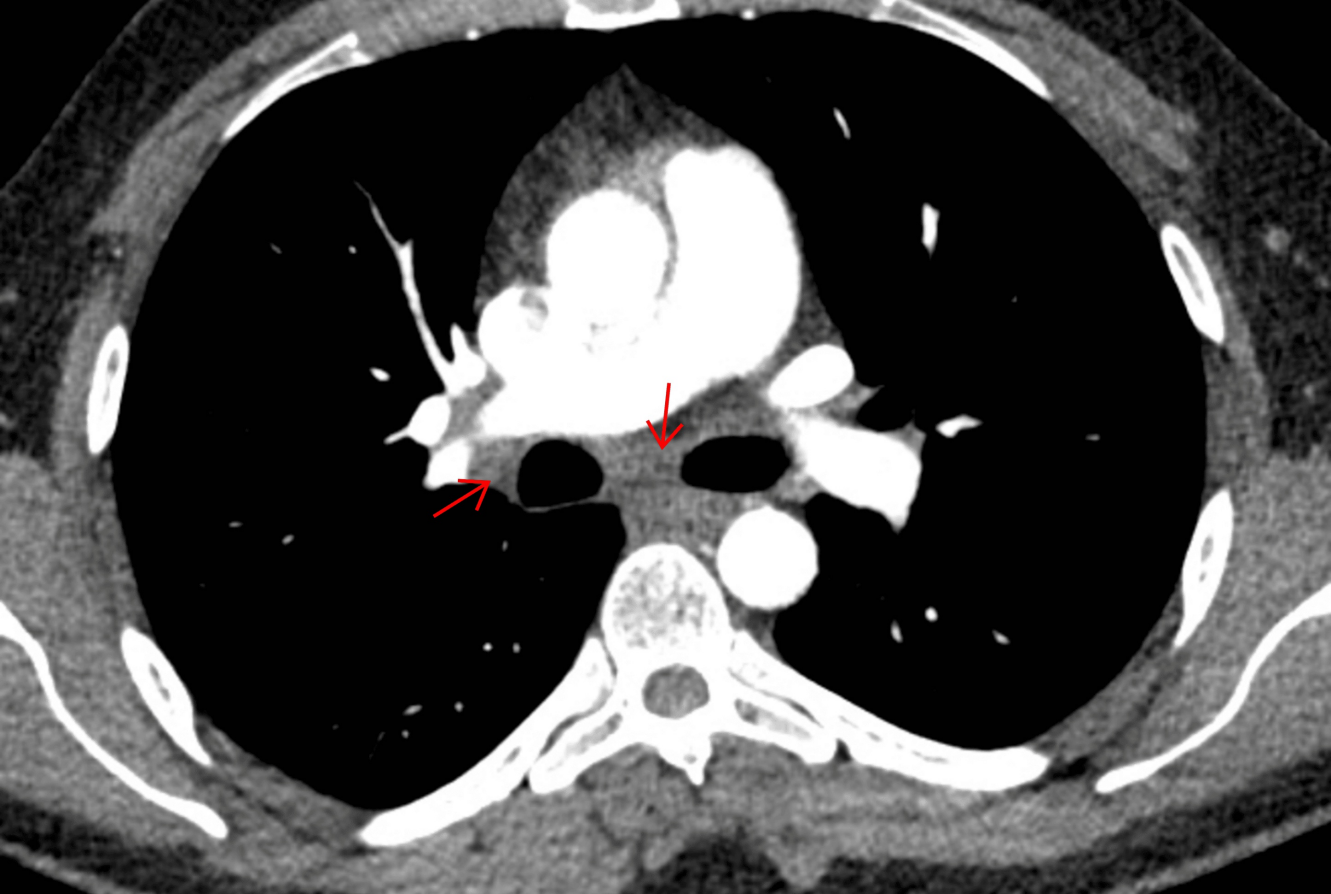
CT thorax showing interval resolution of right hilar and subcarinal lymph node CT: computed tomography
